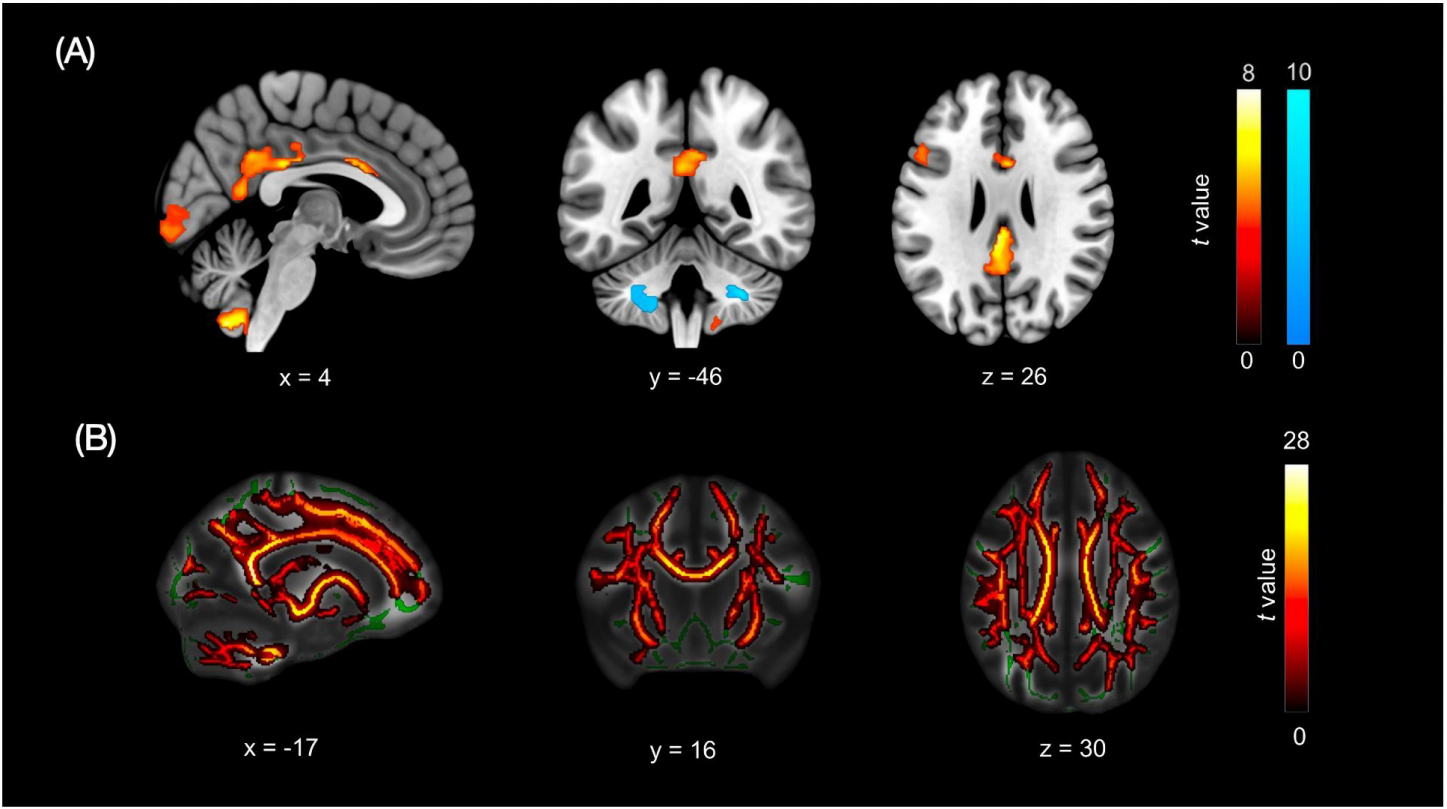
Metabolic and white-matter alterations in pDOC compared with HC. (A) FDG-PET glucose metabolism. Warm clusters correspond to higher uptake in controls, whereas cool clusters correspond to higher uptake in pDOC. (B) TBSS findings. Warm clusters identify regions with lower FA in pDOC.
